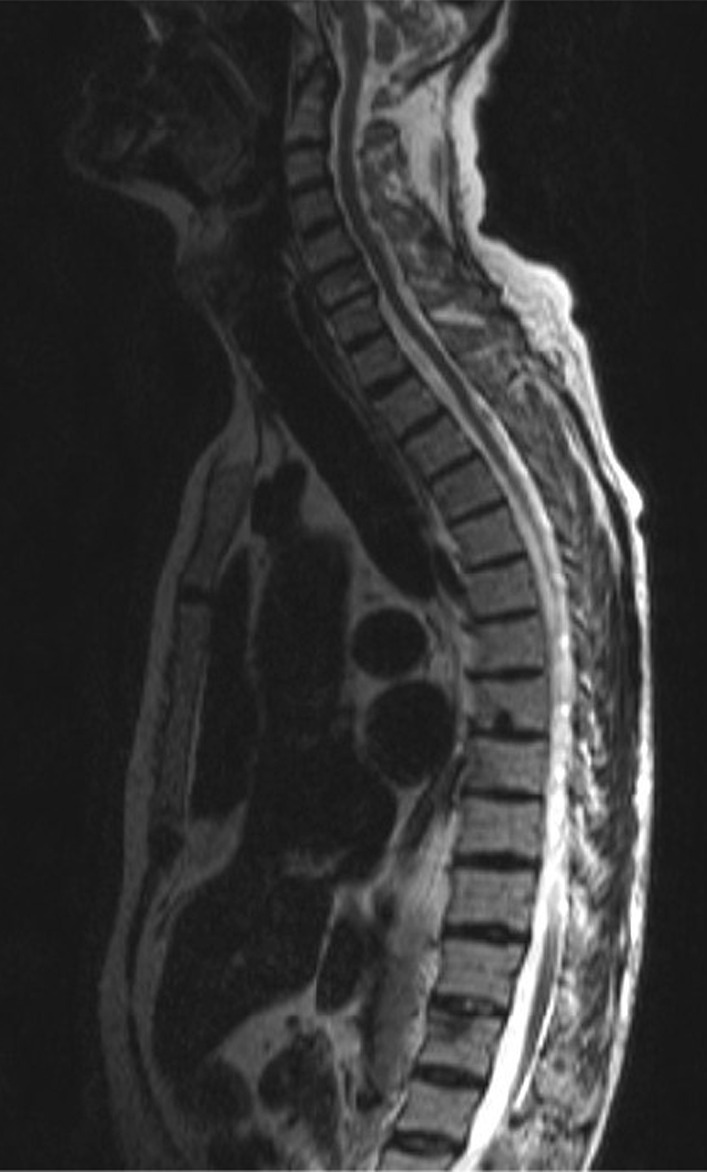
Magnetic resonance imaging of the thoracic spine with multiple bone metastases and no compression of the spinal cord
